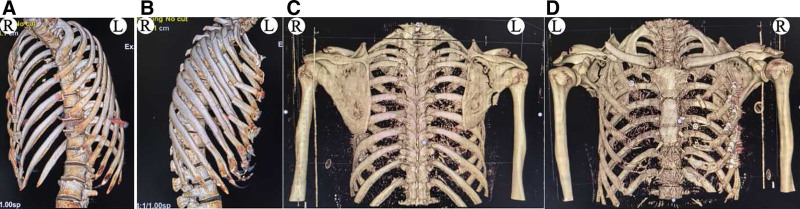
CT scan of chest showing: (A) and (B) bilateral multiple rib fractures; (C) the left scapular fracture; and (D) bilateral clavicle fractures. CT = computed tomography.
